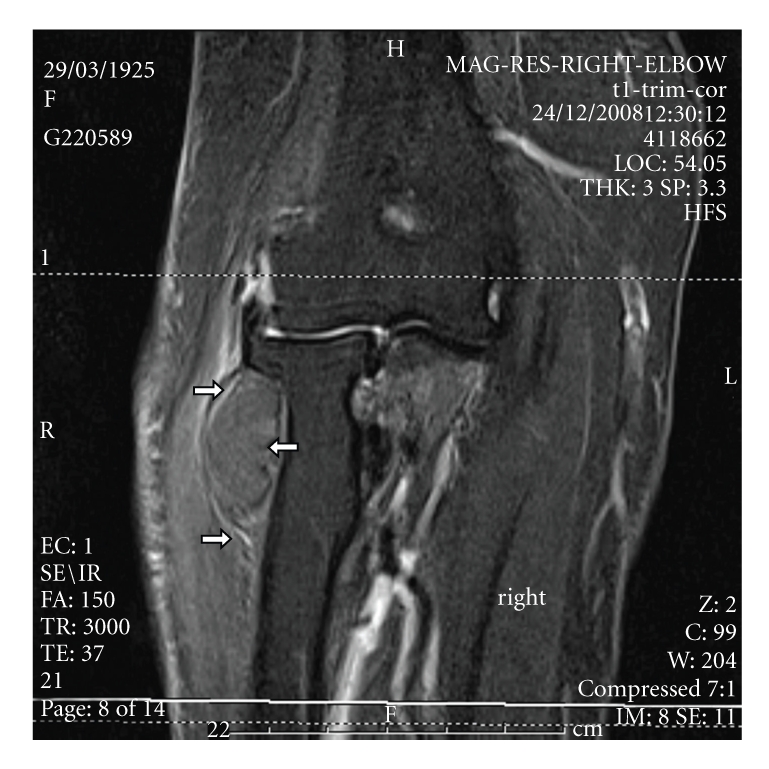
T1-weighted coronal MR image reveals the displacement exerted on surrounding structures by the lesion around the radius, white arrows.
